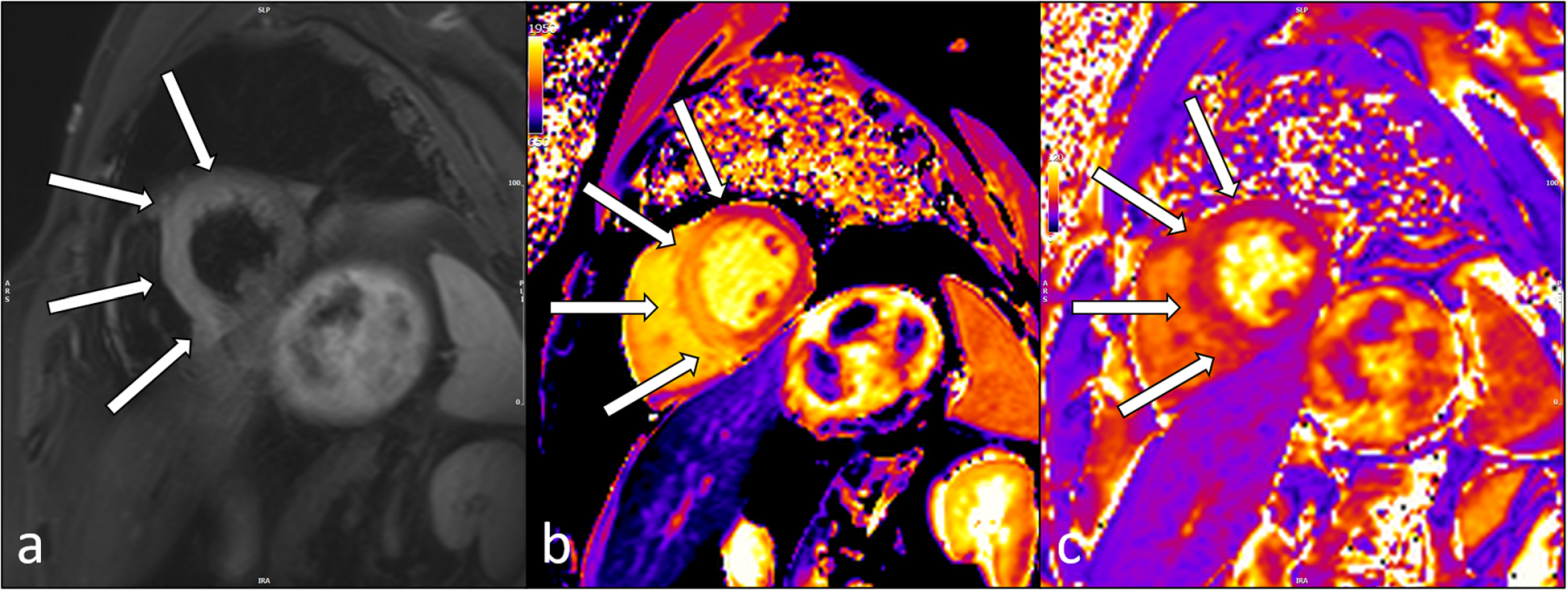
Thirty-two-year-old man with acute anteroseptal myocardial infarction (same patient as in Fig. 1). T2-STIR image shows excessive edema (a, white arrows), corresponding to increased native T1 (b, white arrows) and T2 (c, white arrows) relaxation times in the affected myocardium. T2-STIR, T2-weighted short-tau inversion recovery
